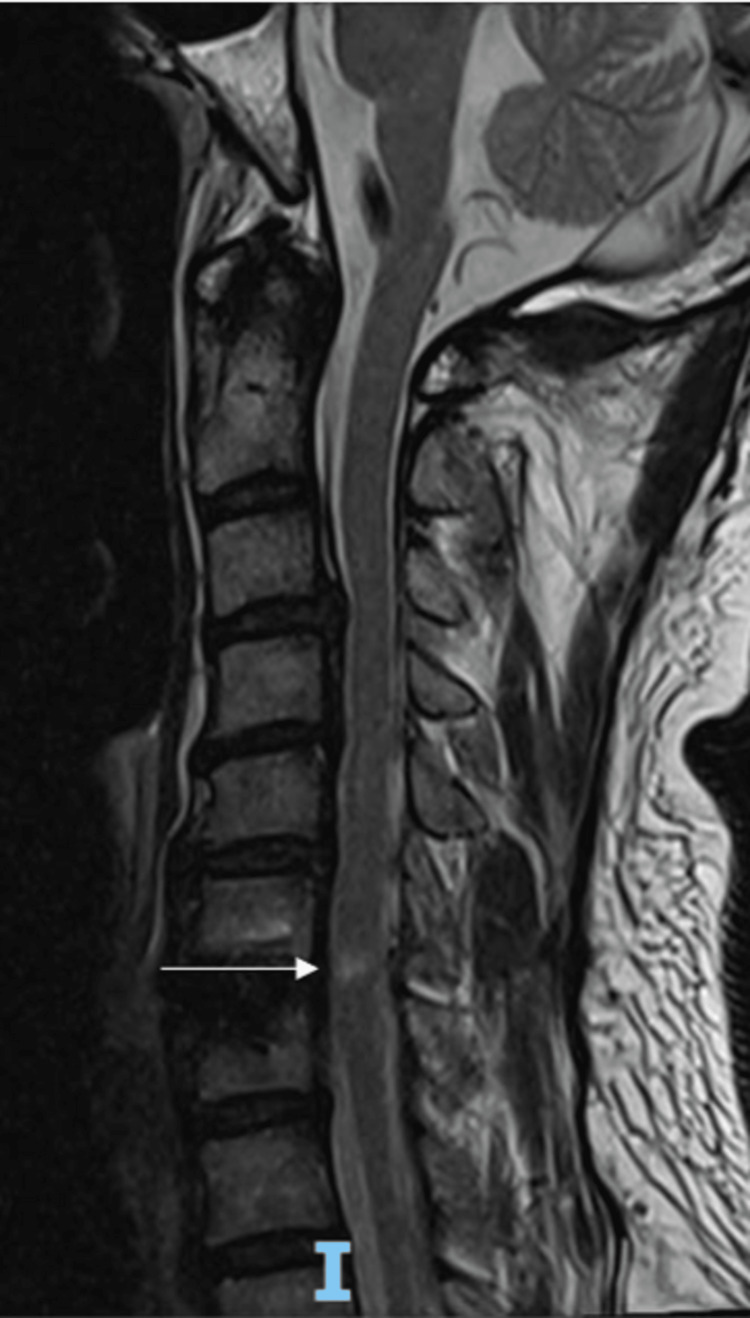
Postoperative T2-weighted MRI of the cervical spine (sagittal view) New focal gliosis/edema within the spinal cord at C6/C7 (arrow) MRI: magnetic resonance imaging
